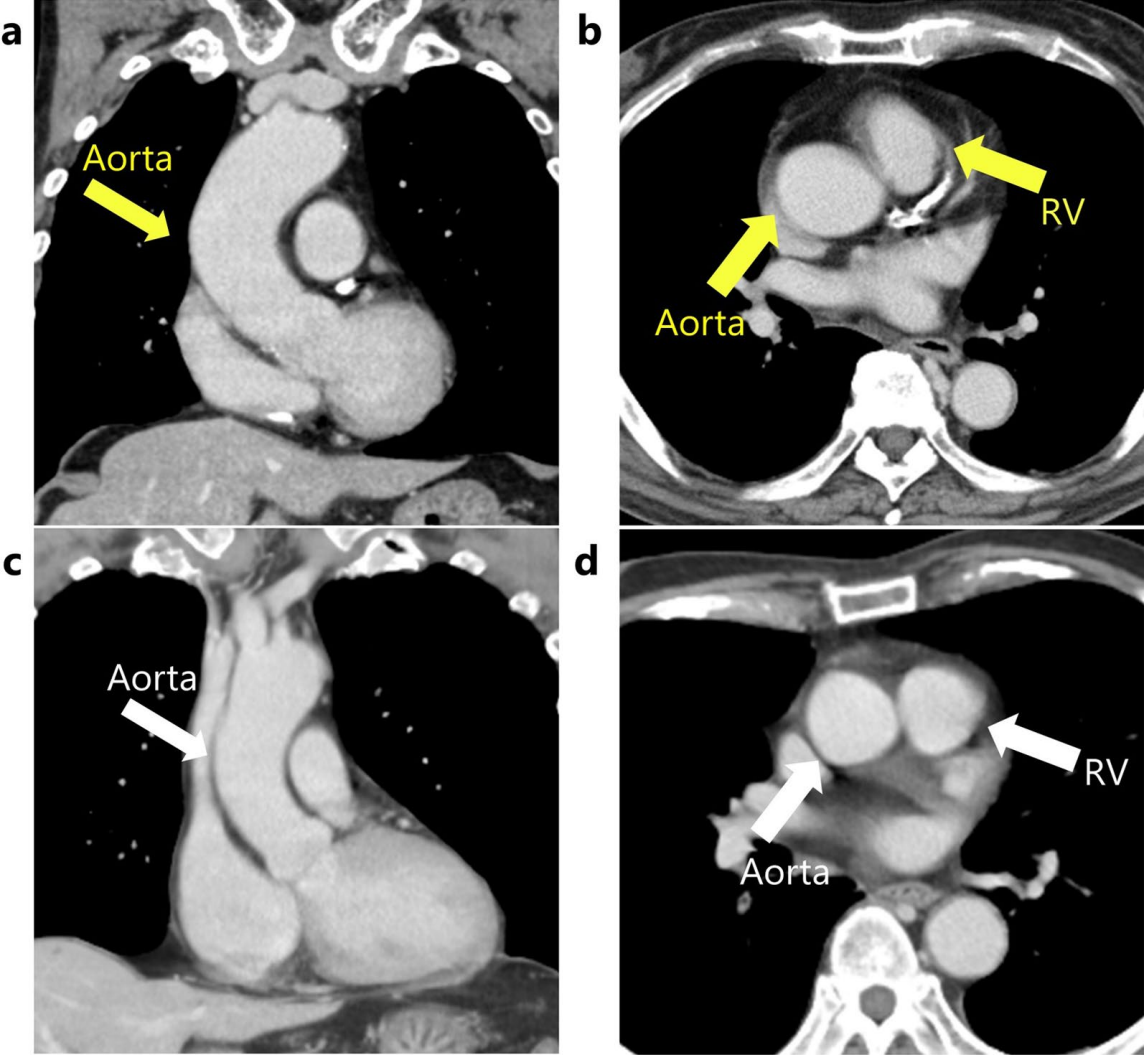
Preoperative computed tomography images. a, b The ascending aorta ran dorsally and more curved to the right than usual, and the right ventricle near the root of the pulmonary artery rose toward the sternum. c, d Images of a normal anatomy. *RV* right ventricle
